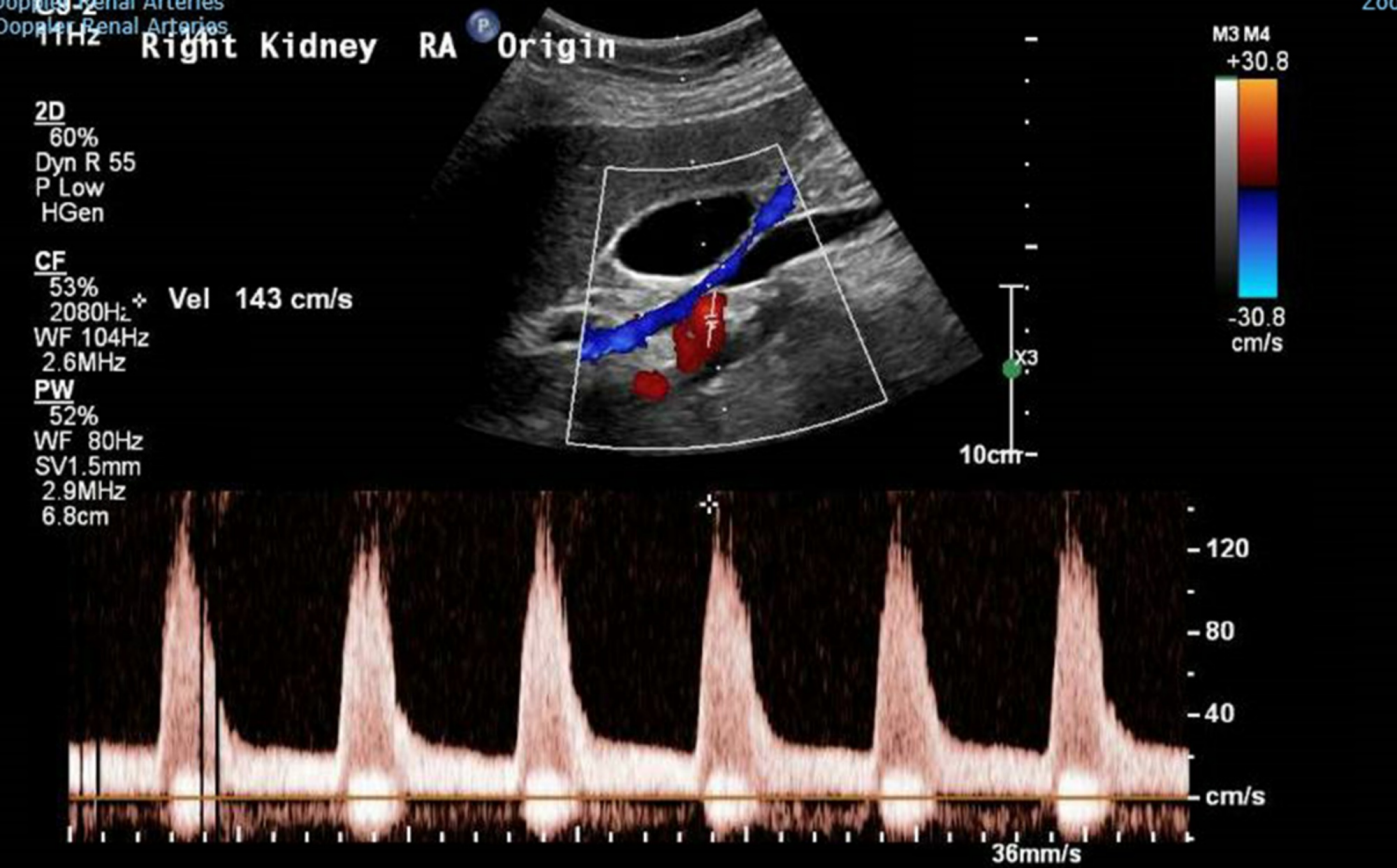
Doppler renal artery showing no intrarenal parvus tardus pattern to suggest renal artery stenosis
